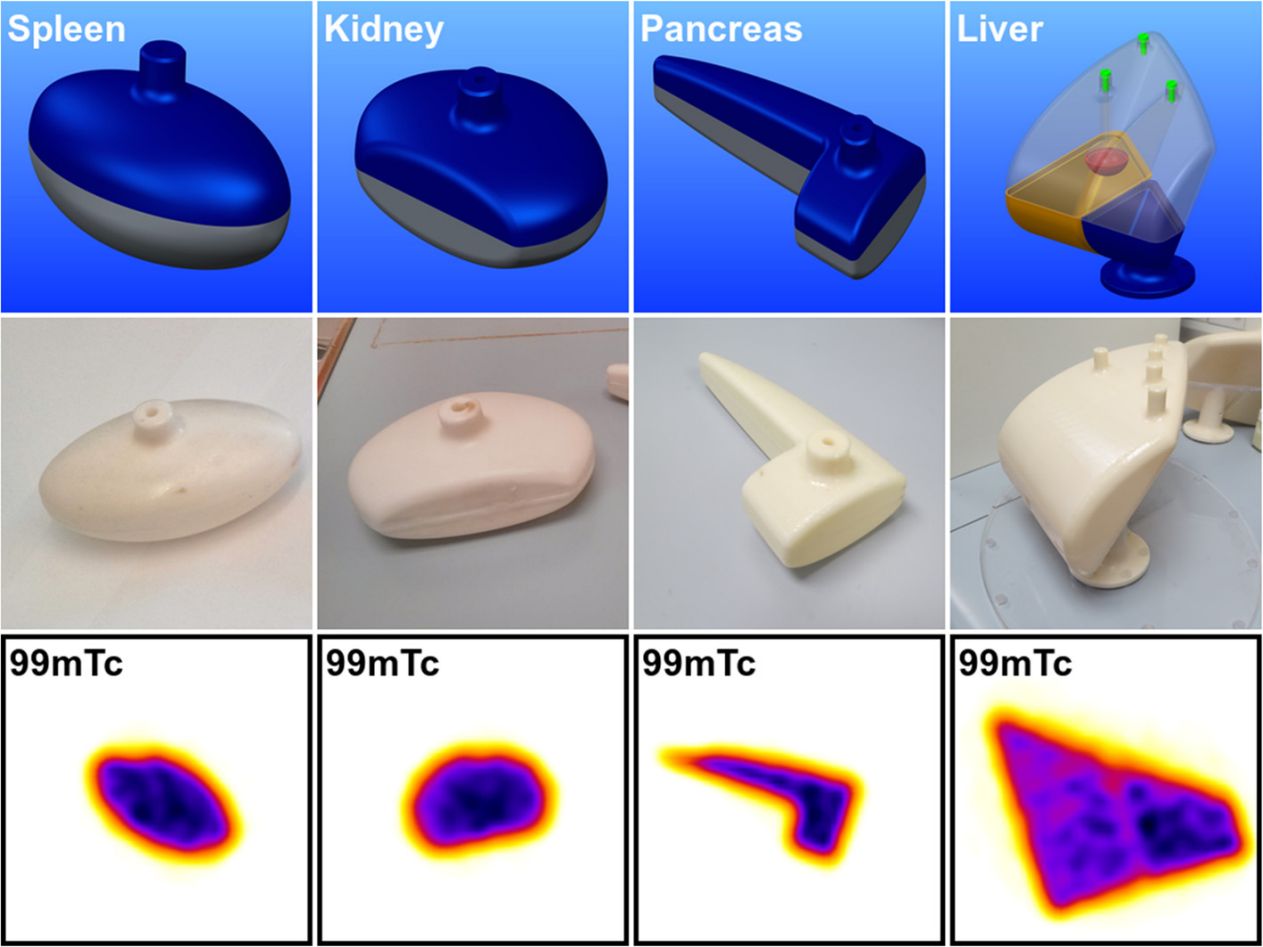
CAD models designed from adult male mathematical models defined in [4] (*top*). The liver model (*right*) has been extended to include a spherical tumour and separate lobes. Organ inserts printed with white ABSplus (*middle*). Central slice through SPECT reconstruction of inserts containing ^99*m*^Tc (*bottom*)
